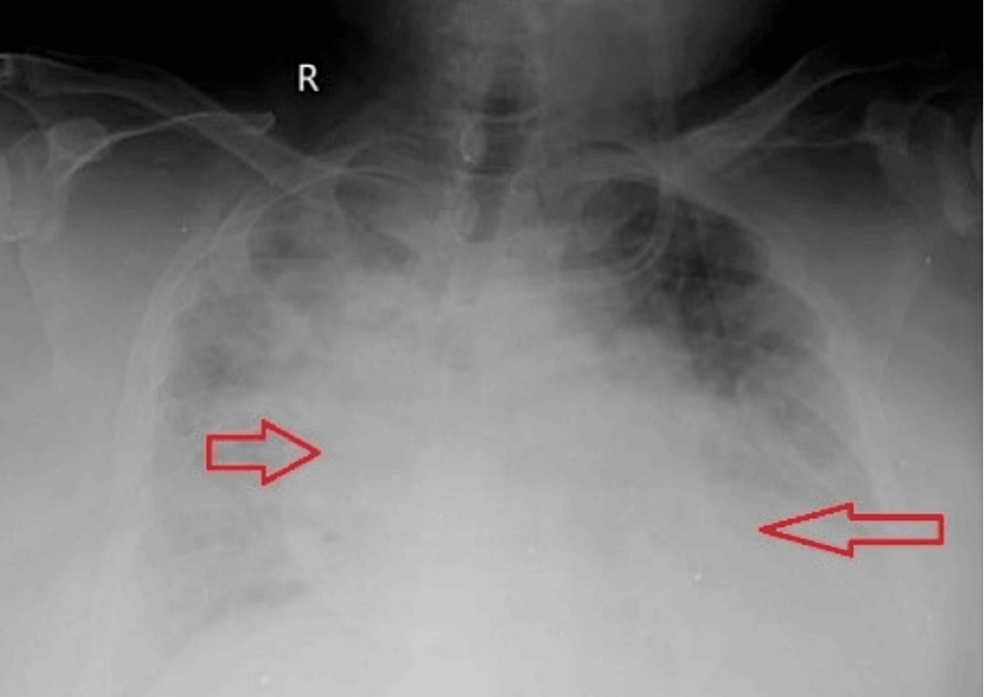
Diffuse bilateral patchy opacities seen on PA view of chest X-ray PA, poster anterior
